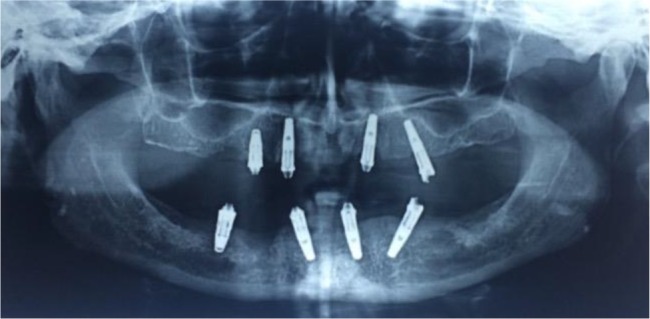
Radiographic view of distal implants replacing the failed previous implants on the left side.
